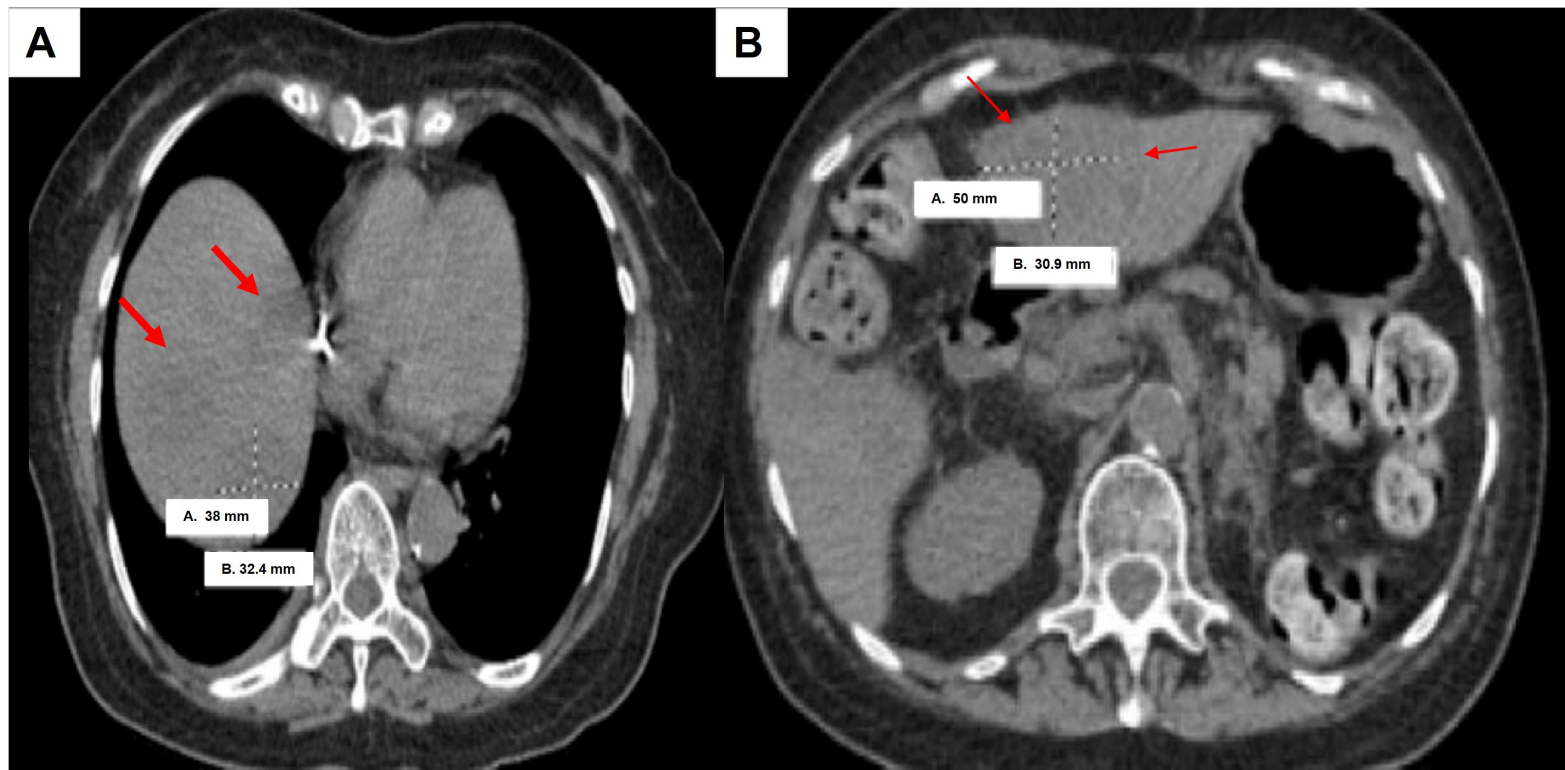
Computed tomography (CT) scan of clinical case 3 showing liver metastases (red arrows) and largest size measuring 38 mm × 32.4 mm
